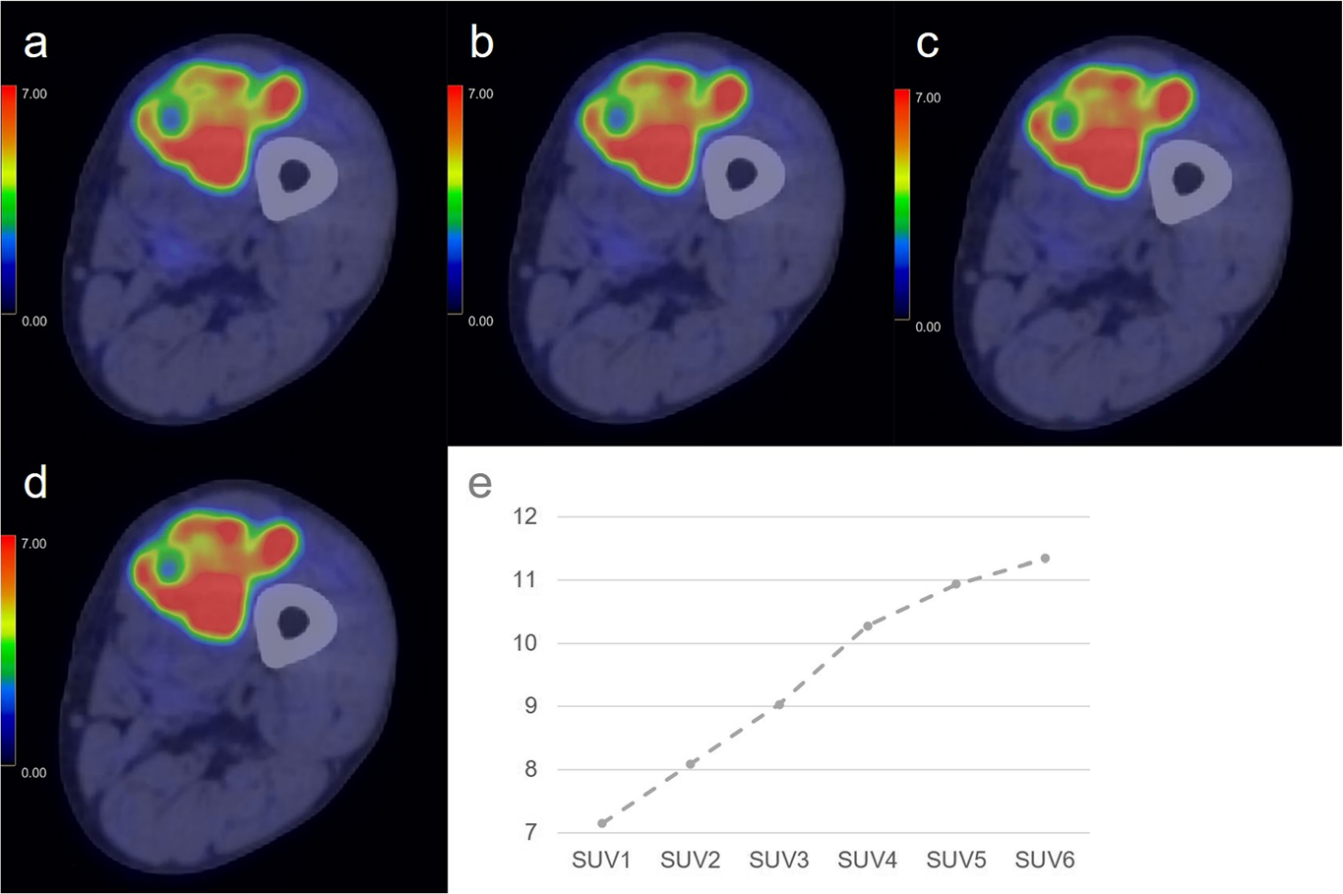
A 78-year-old woman with metastatic soft tissue tumour from gastric cancer in the left thigh. Dynamic positron-emission tomography/computed tomography (PET/CT) (a–d) images are shown. In all dynamic phases (a–d), the soft tissue tumour is avid for methionine (MET). The inhomogeneous and intense MET accumulation in soft tissue tumours is identifiable from the dynamic first phase (SUVmax: dynamic first phase (a), 7.15; dynamic second phase (b), 8.09; dynamic third phase, 9.03; dynamic fourth phase (c), 10.28; dynamic fifth phase, 10.94; dynamic sixth phase (d), 11.34). The accumulation gradually becomes more intense in most parts of the tumour during the dynamic phases (a–d). The time-SUVmax curve (e) shows a high level in SUVmax at the dynamic first phase and a continuous increase during the dynamic phases
